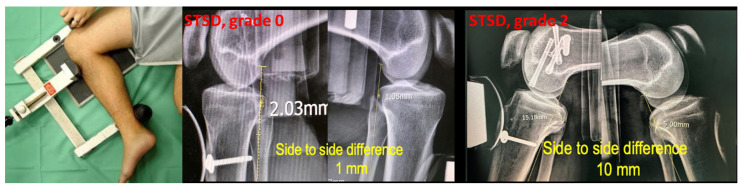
Assessment of posterior instability using lateral stress radiography. Left panel, the patient positioned in the lateral decubitus position for lateral stress radiographic assessment; posterior knee instability Grade 0, STSD in posterior tibial translation of 0–3 mm (middle panel) and Grade 2, 5–10 mm (right panel).
